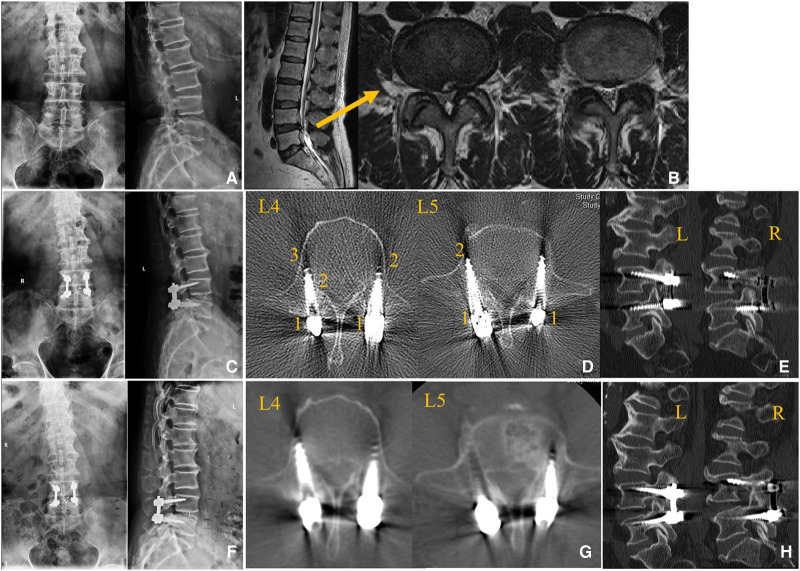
Case of the application of the nomogram. (A,B) Preoperative radiological data. (C–E) Immediate postoperative radiological data. (F–H) Radiological data at 1-year follow-up. (A) Lumbar spine x-ray. (B) Lumbar spine MRI demonstrating lumbar stenosis at L4/5. (C) Postoperative lumbar spine x-ray. (D) Postoperative lumbar spine CT scan indicating that the CBCLs of L4L, L4R, L5L, and L5R were 2, 3, 1, and 2, respectively. (E) Sagittal view of the lumbar spine CT scan. (F) After 1-year follow-up, a lumbar x-ray demonstrated a lucent zone at L4R and L5R. (G) Lumbar spine CT scan showing obvious screw loosening at L4L, L5L, and L5R. (H) Sagittal view of the lumbar spine CT scan.
